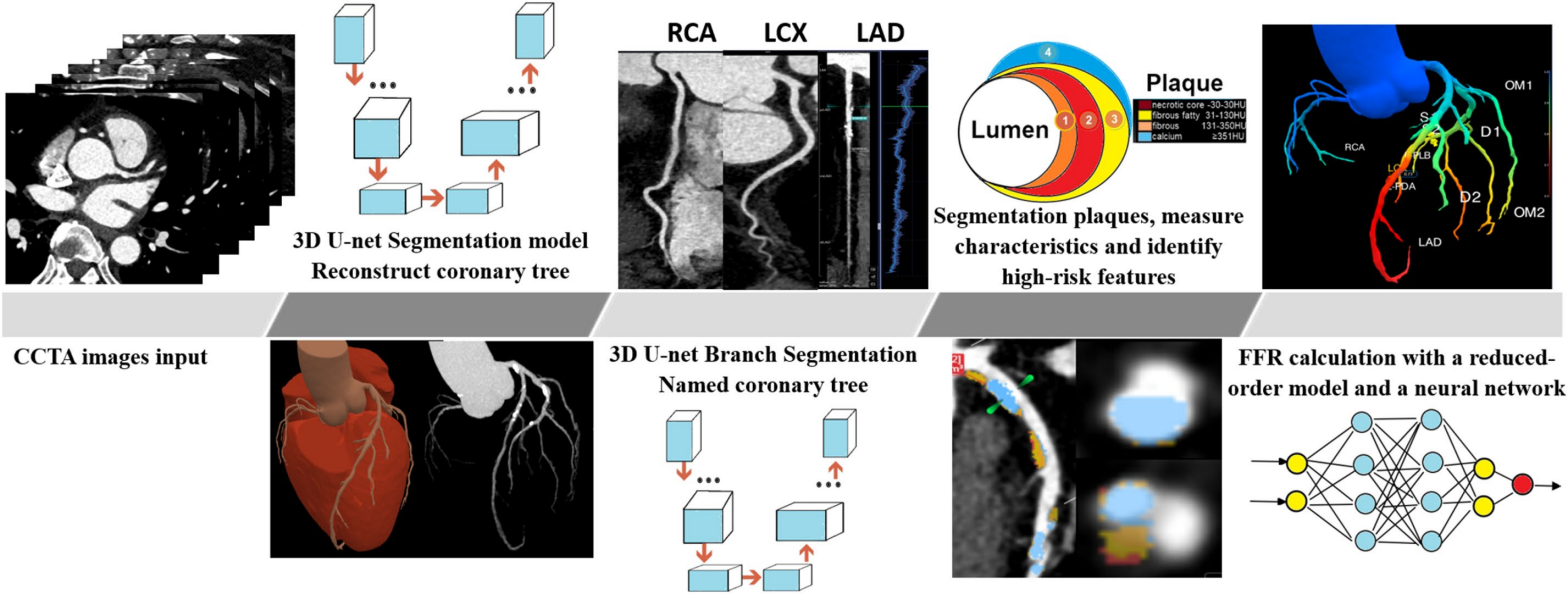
Principle of the fully-automatic plaque characteristics and FFR-CT calculation by AI software.
